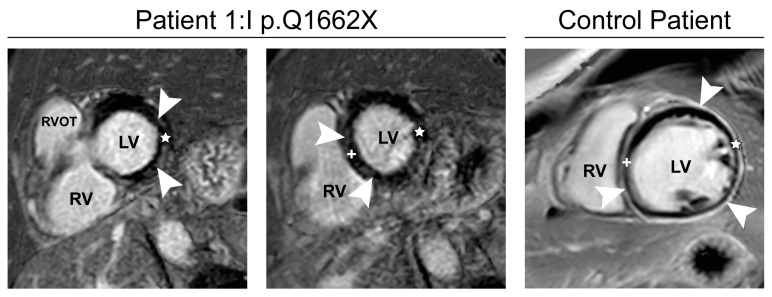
Cardiac MRI. Phase-sensitive inversion-recovery (PSIR) of patient I:1, Basal (left) and mid-short-axis (middle) images show subepicardial to mid-wall late enhancement (arrowheads) in the lateral wall (star) and interventricular septum (+). Typical example late gadolinium enhancement image with diffuse late enhancement in a 23-year-old control patient with FLNC-cardiomyopathy (p.E2009GfsX29; (right), arrowheads). RVOT: Right ventricular outflow tract, LV: Left ventricle, RV: Right ventricle.
